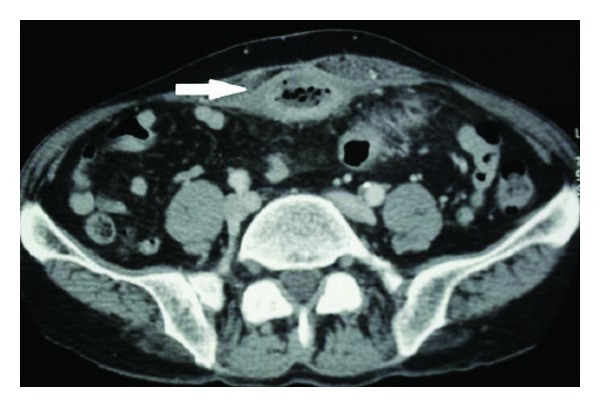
CT showed an air-fluid collection in the urachus (white arrow).
